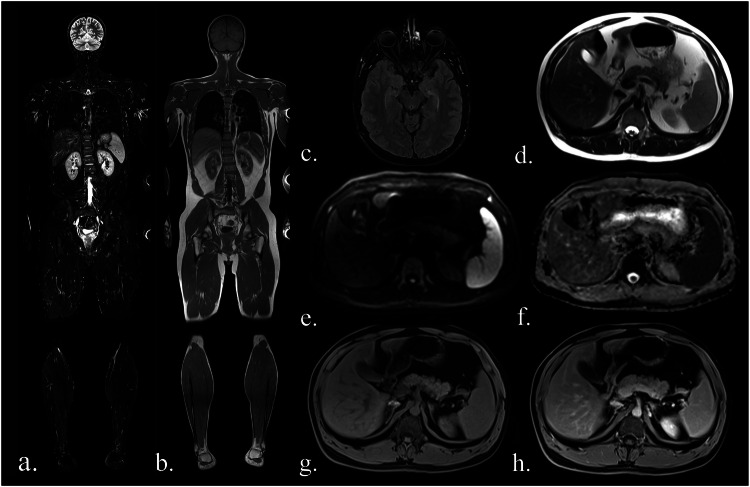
Sequences included in the study MRI protocol: (a) coronal TIRM (turbo inversion recovery magnitude) sequence, (b) coronal T1-weighted sequence, (c) axial FLAIR (fluid-attenuated inversion recovery) sequence (head only), (d) axial T2-weighted fast spin-echo sequence (HASTE; Siemens), (e) axial diffusion-weighted imaging (b-value 900), (f) an axial ADC map, (g) axial T1-Dixon water-only images before contrast agent and (h) after administration of contrast agent. Images were cropped to allow all sequences to be displayed
